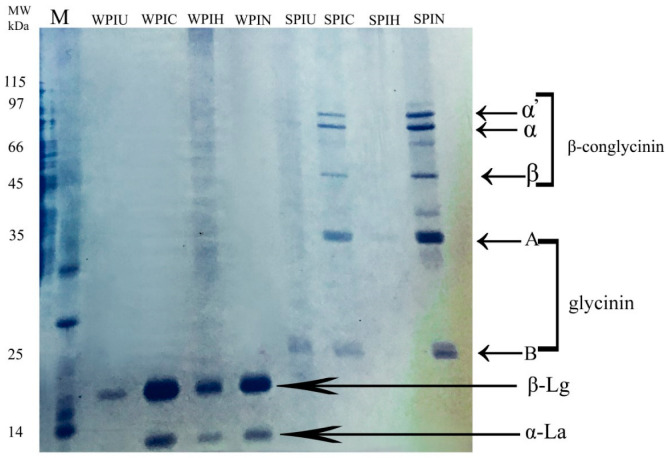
Visual appearance and SDS-PAGE patterns of SPI and WPI. Lane M: marker protein band. Lane MW: kDa molecular weight of marker. Lane WPIU: whey protein isolates ultrasonic treatment band. Lane WPIC: whey protein isolates cold plasma treatment band. WPIH whey protein heat treatment band. Lane WPIN: natural whey protein isolate. Lane SPIU: soy protein isolates ultrasonic treatment. Lane SPIC: soy protein isolates cold plasma treatment. Lane SPIH: soy protein isolate heat treatment. Lane SPIN: natural soy protein isolate. Soy protein isolates ά, α, and β subunits of β-conglycinin and the acidic and basic subunits of glycinin. Whey protein isolates α-lactalbumin (α-La) and β-lactoglobulin (β-Lg).
